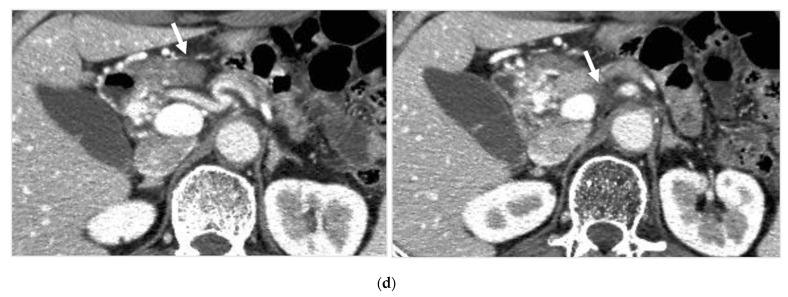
Locally advanced cancer of the pancreas with excellent treatment compliance. A 78-year-old man with locally advanced pancreatic carcinoma at pancreatic body received 4 treatment courses in the first year, resulting in CR and continued life for 144 months after diagnosis. (a) Postcontrast CT (arterial phase) immediately before the first treatment course. A mass of low attenuation with maximal diameter of 3.5 cm (arrows) was observed at the proximal portion of the pancreatic body occluding splenic vein and extending to the common hepatic artery, splenic artery, and superior mesenteric artery. (b) The first arterial administration of chemotherapeutic agents (mitomycin C and epirubicin hydrochloride) mixed with contrast media after 3 weekly intravenous low-dose gemcitabine. Selective dorsal pancreatic angiography shows occlusion of the distal dorsal pancreatic artery (arrow) and severe encasement of its branches depicting a hypovascular mass (short arrows). (c) CT taken during dorsal pancreatic angiography show a dense opacification of most parts of the tumor (arrows). Note opacification of wall of common hepatic artery (short arrow). Twelve milliliters of contrast media (20 mL) mixed with chemotherapeutic agents were administrated at the dorsal pancreatic artery. Two milliliters of a mixture of contrast media and chemotherapeutic agents were also administrated at the pancreatica magna artery (not shown). (d) Postcontrast CT (arterial phase) after two treatment courses showed a marked decrease in the size of the tumor (arrow), which had no enhancement effect until the parenchymal phase. Note the atrophy of the pancreatic body and the tail and splenic vein. After this arterial administration, all elevated tumor markers became normal, including CA19-9 (142.7 U/mL → 7.9 U/mL), elastase-1 (1340 ng/dl → 121 ng/dL), and SPAN-1 (110 U/mL → 5.7 U/mL). The patient showed no sign of recurrence for 11 years.
